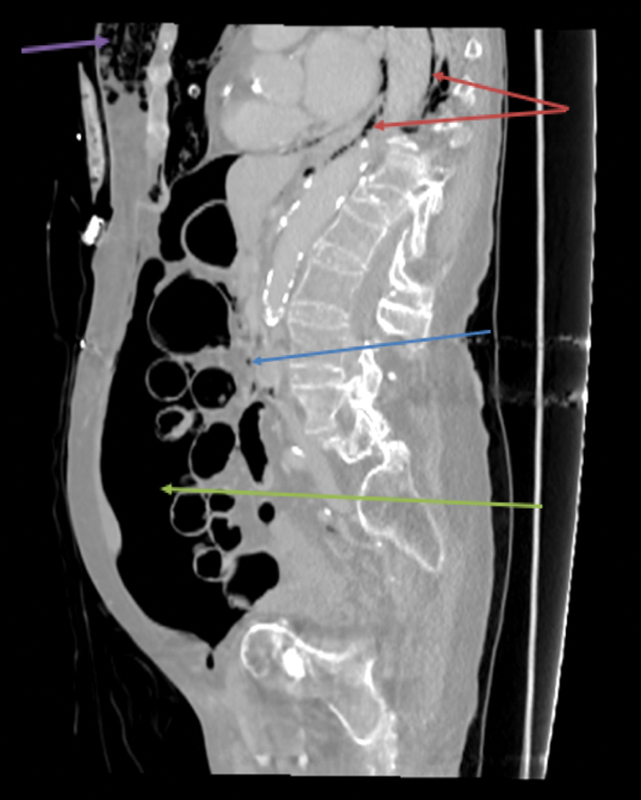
Sagittal computed tomography image demonstrating pneumoperitoneum (green arrow), air in retroperitoneum (blue arrow) and alongside aorta (red arrows) and subcutaneous emphysema in the anterior chest wall (purple arrow).
